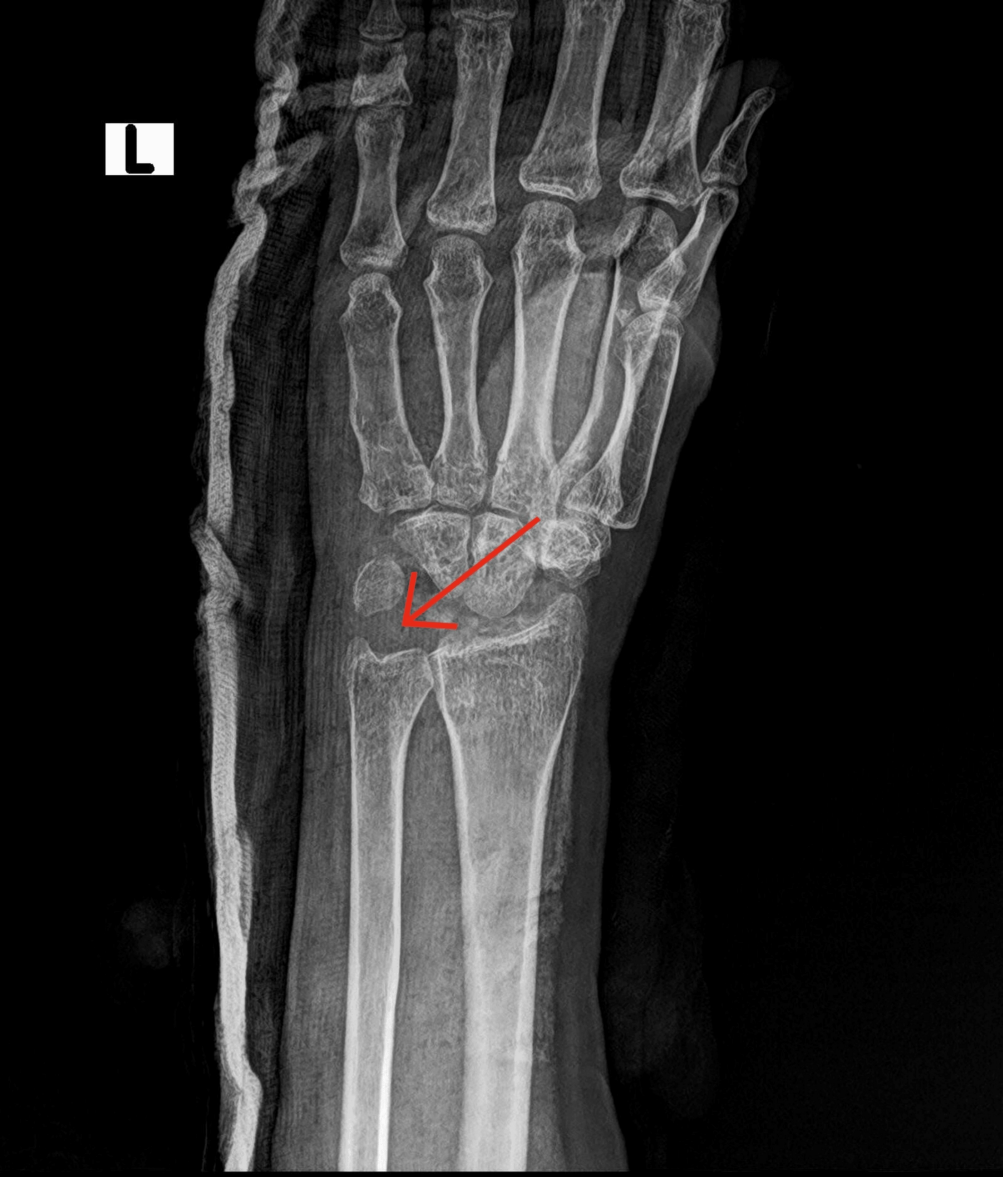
Post-operative radiological findings The plain X-ray in the AP view shows the absence of the proximal carpal bones, indicating a probable partial carpectomy.
